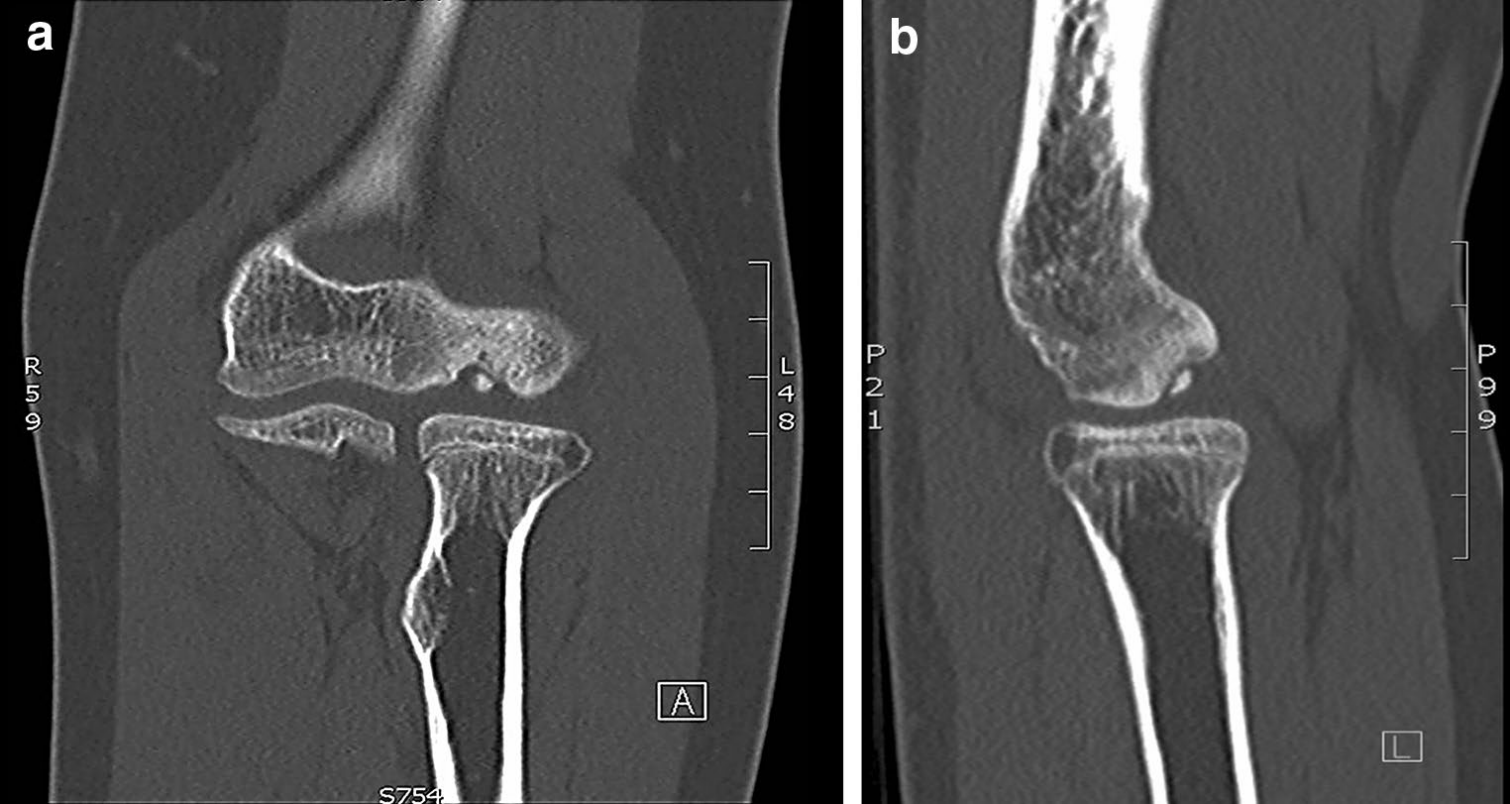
Coronal (a) and sagittal (b) CT-scan images of an osteochondral defect of the capitellum of the *right* elbow in a young girl with osteochondritis dissecans
